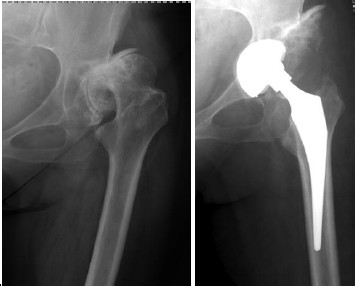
Radiograph of dysplastic hip that dislocated once.
